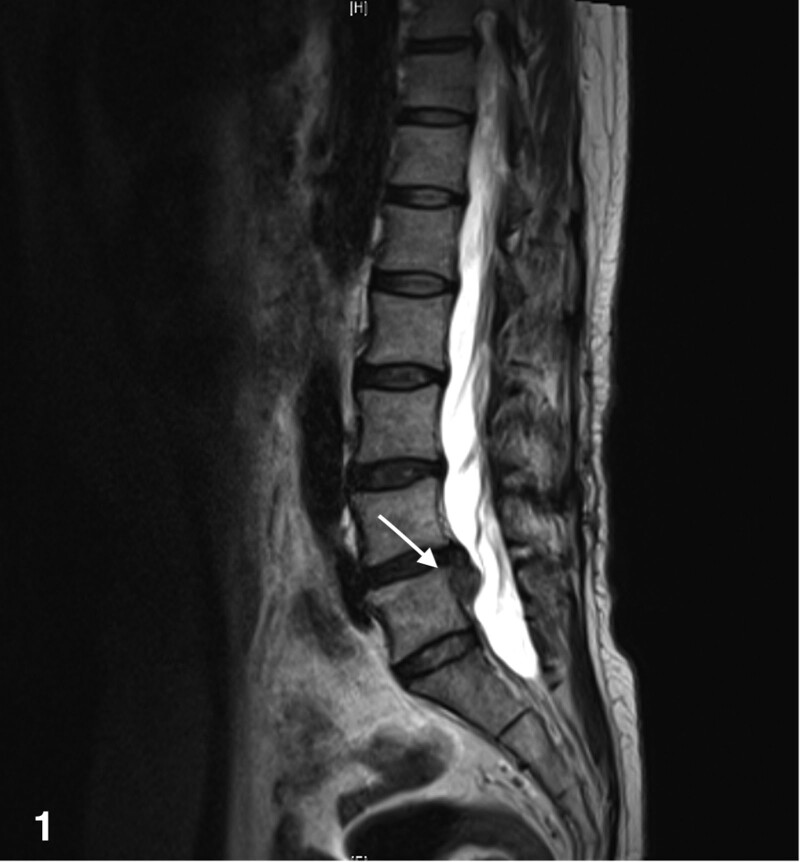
Sagittal MRI of lumbar spine in patients before UBE surgery. MRI = magnetic resonance imaging.
